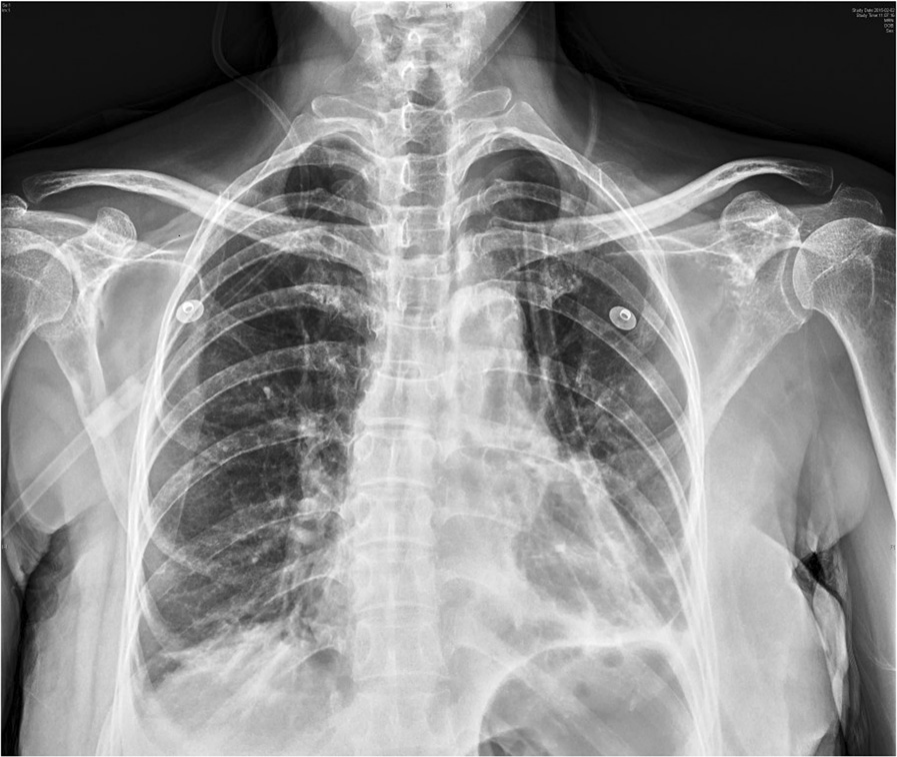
Chest X-ray of the 1st postoperative day
